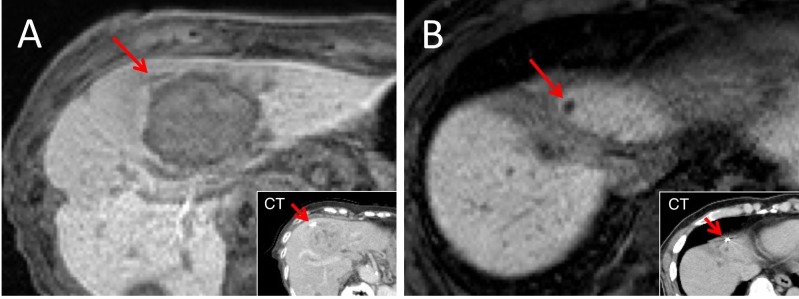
Various forms of the GA in CT and MRI images. These images show the Gold Anchor^TM^ (GA) in contrast-enhanced computed tomography (CT) and gadolinium-ethoxybenzyl-diethylenetriamine pentaacetic acid (Gd-EOB-DTPA)-enhanced magnetic resonance imaging (MRI) images. A. The GA was placed close to the tumor in a linear shape. B. The GA was placed near the postoperative site in a folded shape. GA = Gold Anchor^TM^
